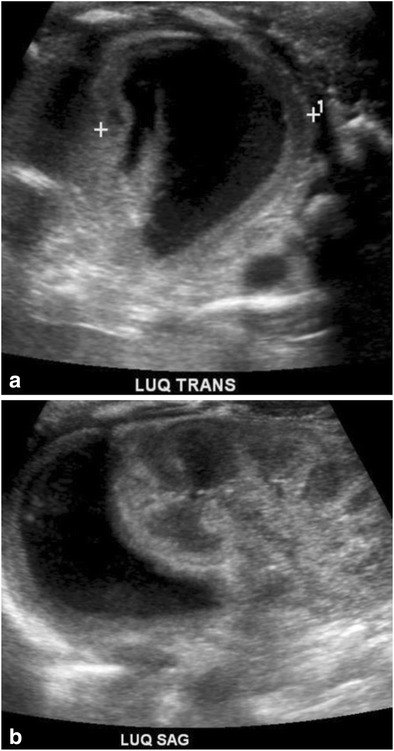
Visceral Injury Ultrasound of the left upper quadrant performed in a 3-day-old revealed a crescentic, near anechoic collection in the location of the left adrenal. Baby had a history of difficult birth characterized by prolonged 2nd stage of labor. Hematoma was followed by serial ultrasound and resolved at 13 weeks of age
